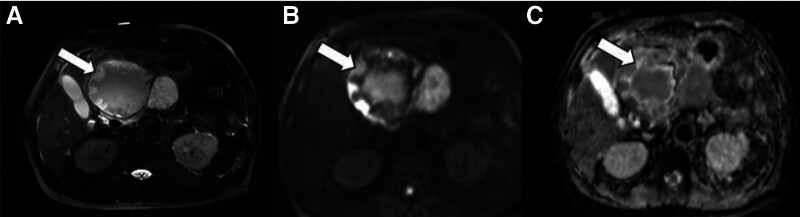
T2-weighted MRI abdomen (A) showing the lesion slightly hyperintense signal, with extensive nodule-like areas of high signal beneath the cyst wall. Diffusion-weighted imaging (B) and apparent diffusion coefficient (C) showing restricted diffusion (white arrow). The cystic fluid also shows restricted diffusion, accompanied by thicker septa without restricted diffusion. MRI = magnetic resonance imaging.
